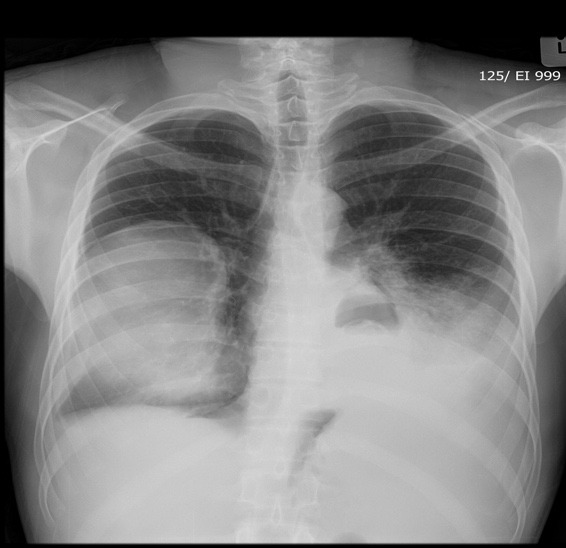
Chest radiograph at presentation
